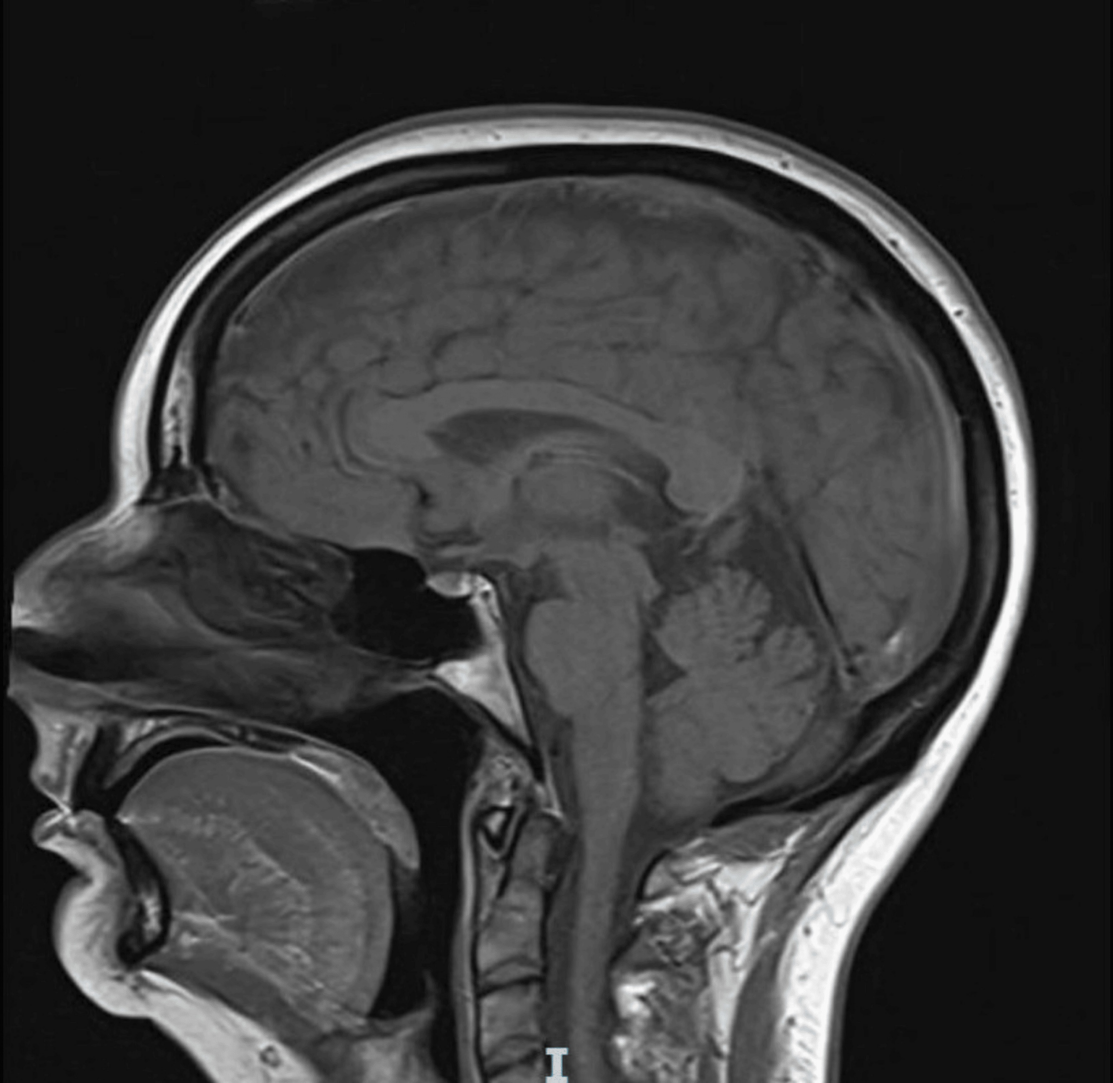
Magnetic resonance imaging (MRI) of the brain (sagittal T1-weighted image) demonstrating no acute intracranial abnormalities.
